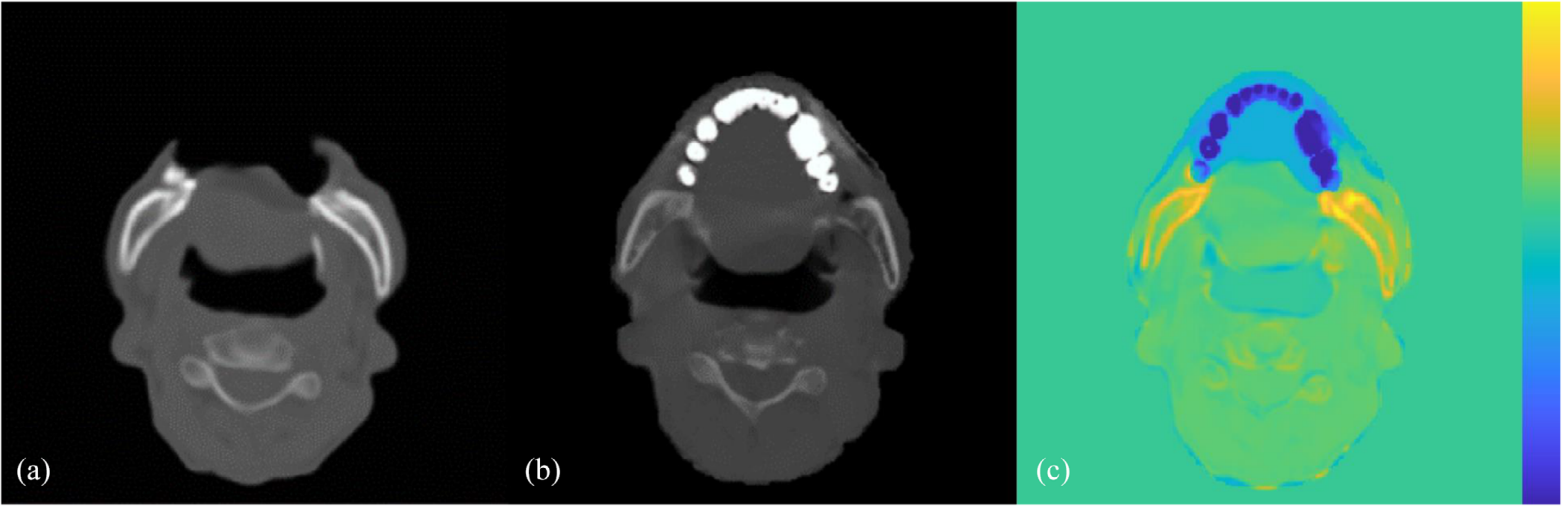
Axial image for subject with fixed denture. This showed the effect of susceptibility artifact to synthetic CT generation. The resampled MRCAT (a) was subtracted with planning CT (b) to obtain subtraction map (c) with yellow and blue colour contributed from resampled MRCAT and planning CT respectively.
